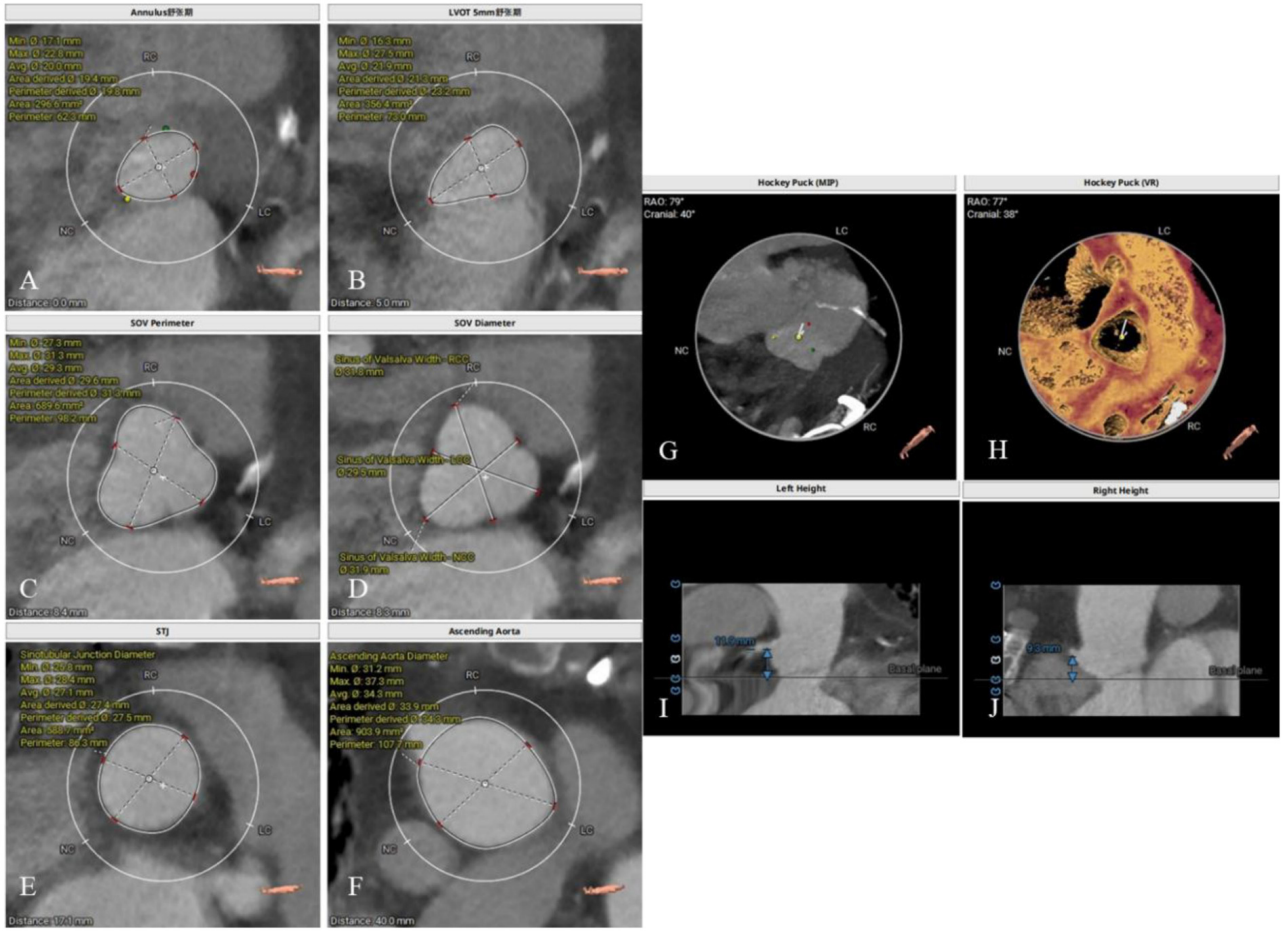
Preoperative CT evaluation. (A) Annular circumference diameter: 19.8 mm; (B) outflow tract circumference diameter: 23.2 mm; (C) sinus of valsalva circumference diameter: 31.3 mm; (D) sinus of valsalva diameter: 31.8 mm, 29.5 mm, 31.9 mm; (E) sinutubular junction circumference diameter: 27.5 mm; (F) aortic circumference diameter 4 cm above the annulus: 34.3 mm; (G,H) no significant calcification observed in the annulus and leaflets; (I) height of the left coronary artery ostium: 11.9 mm; (J) height of the right coronary artery ostium: 9.3 mm.
